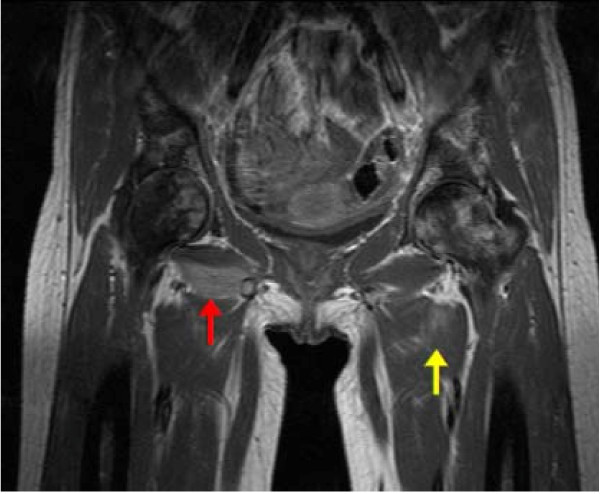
Coronal T1-weighted MRI of the pelvis and hips - initial imaging. Left obturator externus and adductor brevis muscles (yellow arrow), and the right obturator externus, iliacus and iliopsoas muscles (red arrow).
